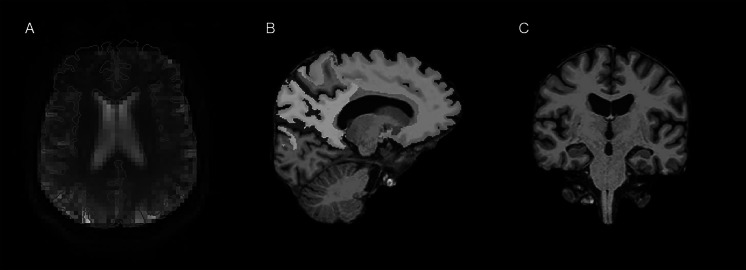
Regions of interest for k_w_ maps. Regions of interest (ROIs) for k_w_ analyses displayed on a representative participant. A The diffusion prepared arterial spin labeling (DP-pcASL) M0 image with the T1-weighted image (red outline) aligned. B The frontal lobar (green), parietal lobar (yellow), and portions of precuneus (blue) masks on a mid-sagittal slice of the T1-weighted image. C The hippocampal (red) mask on a coronal slice of the T1-weighted image. The masks displayed in B and C were then resampled to the resolution of the M0 image
